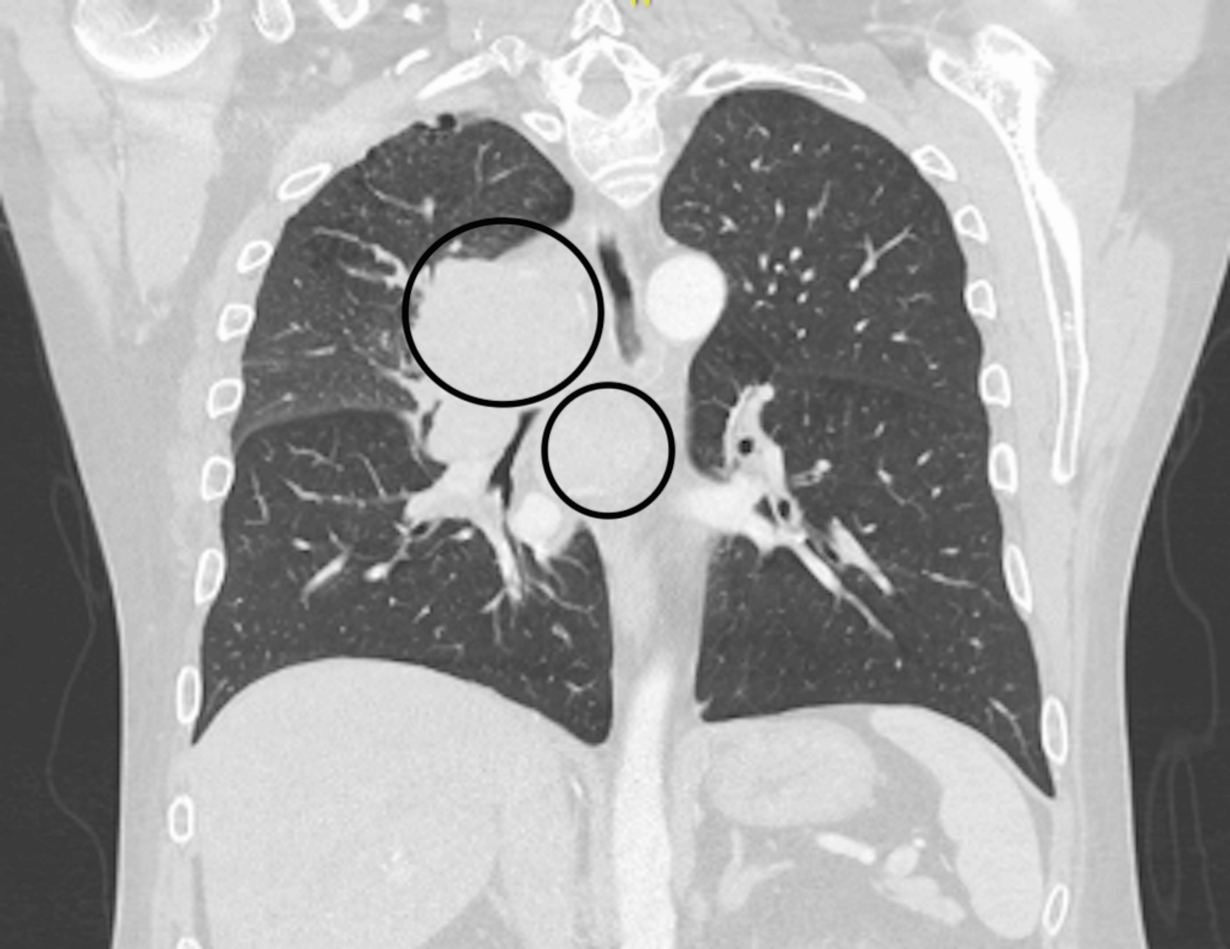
CT chest showing bulky right hilar and subcarinal lymphadenopathy. The right hilar mass and subcarinal lymphadenopathy have been marked using open circles.
